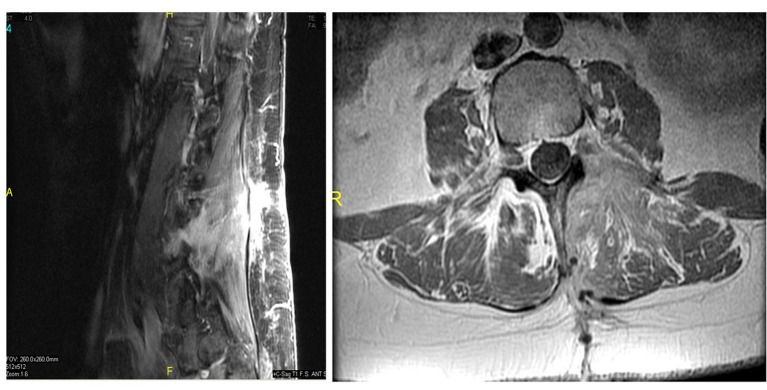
Postoperative T1 sagittal (right) and axial (left) MRI with contrast demonstrating resection of the nerve root sheath tumor.
